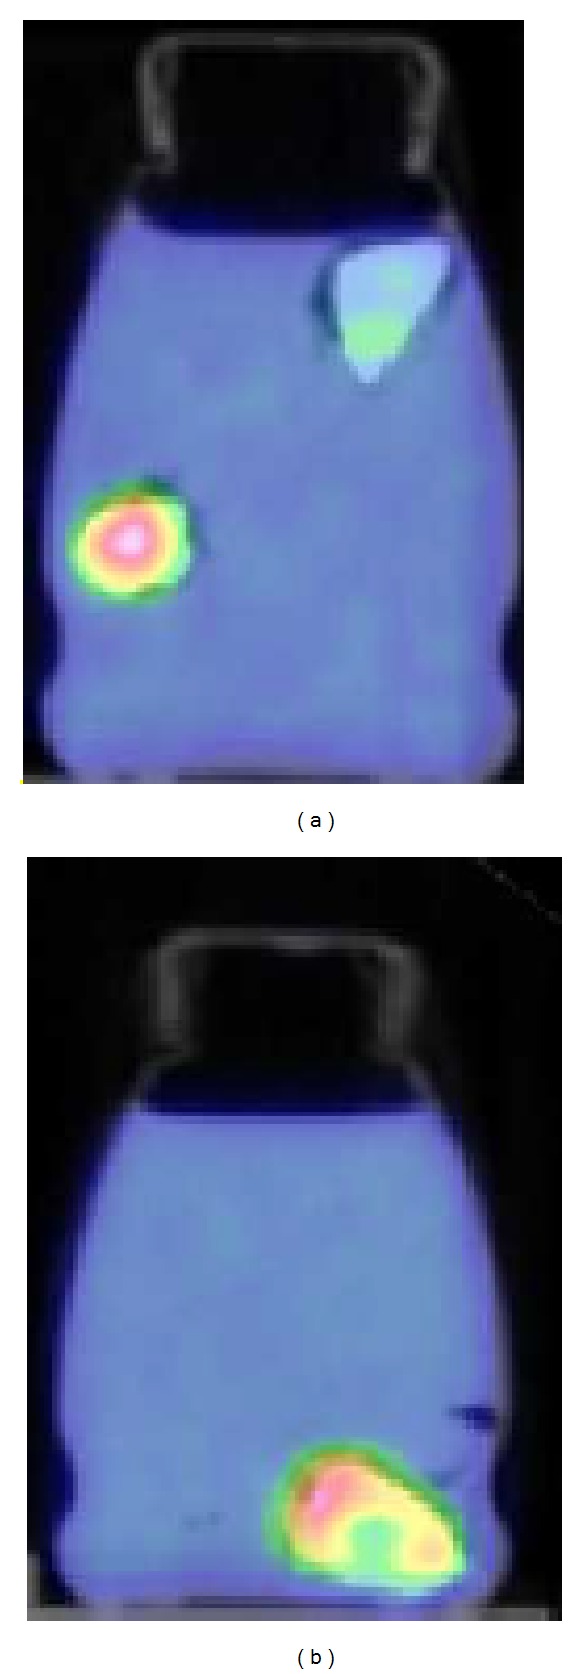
PET-CT images for the oncological nonspherical lesions (zeolites).
